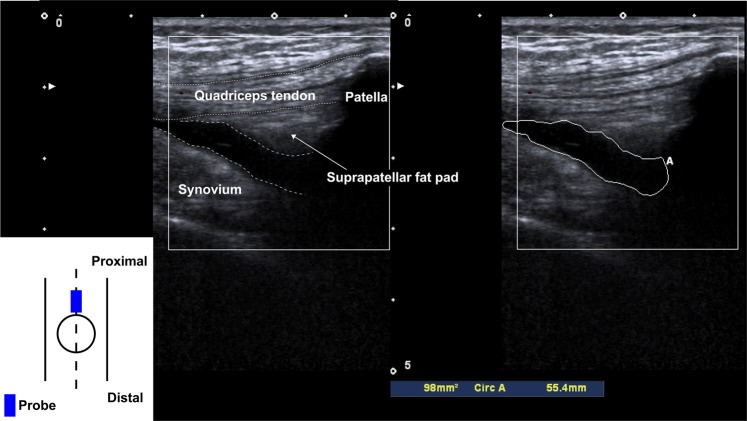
Quantitative evaluation of the suprapatellar effusion area by ultrasonography The ultrasound probe is longitudinally placed at the center at the proximal pole of the patella. Based on the acquired sagittal image, the boundary of echo-free space, which corresponds to the suprapatellar pouch, is traced and the area of suprapatellar effusion (mm^2^) is automatically calculated. Synovial tissue and suprapatellar fat pad are excluded from the current measurement area.
